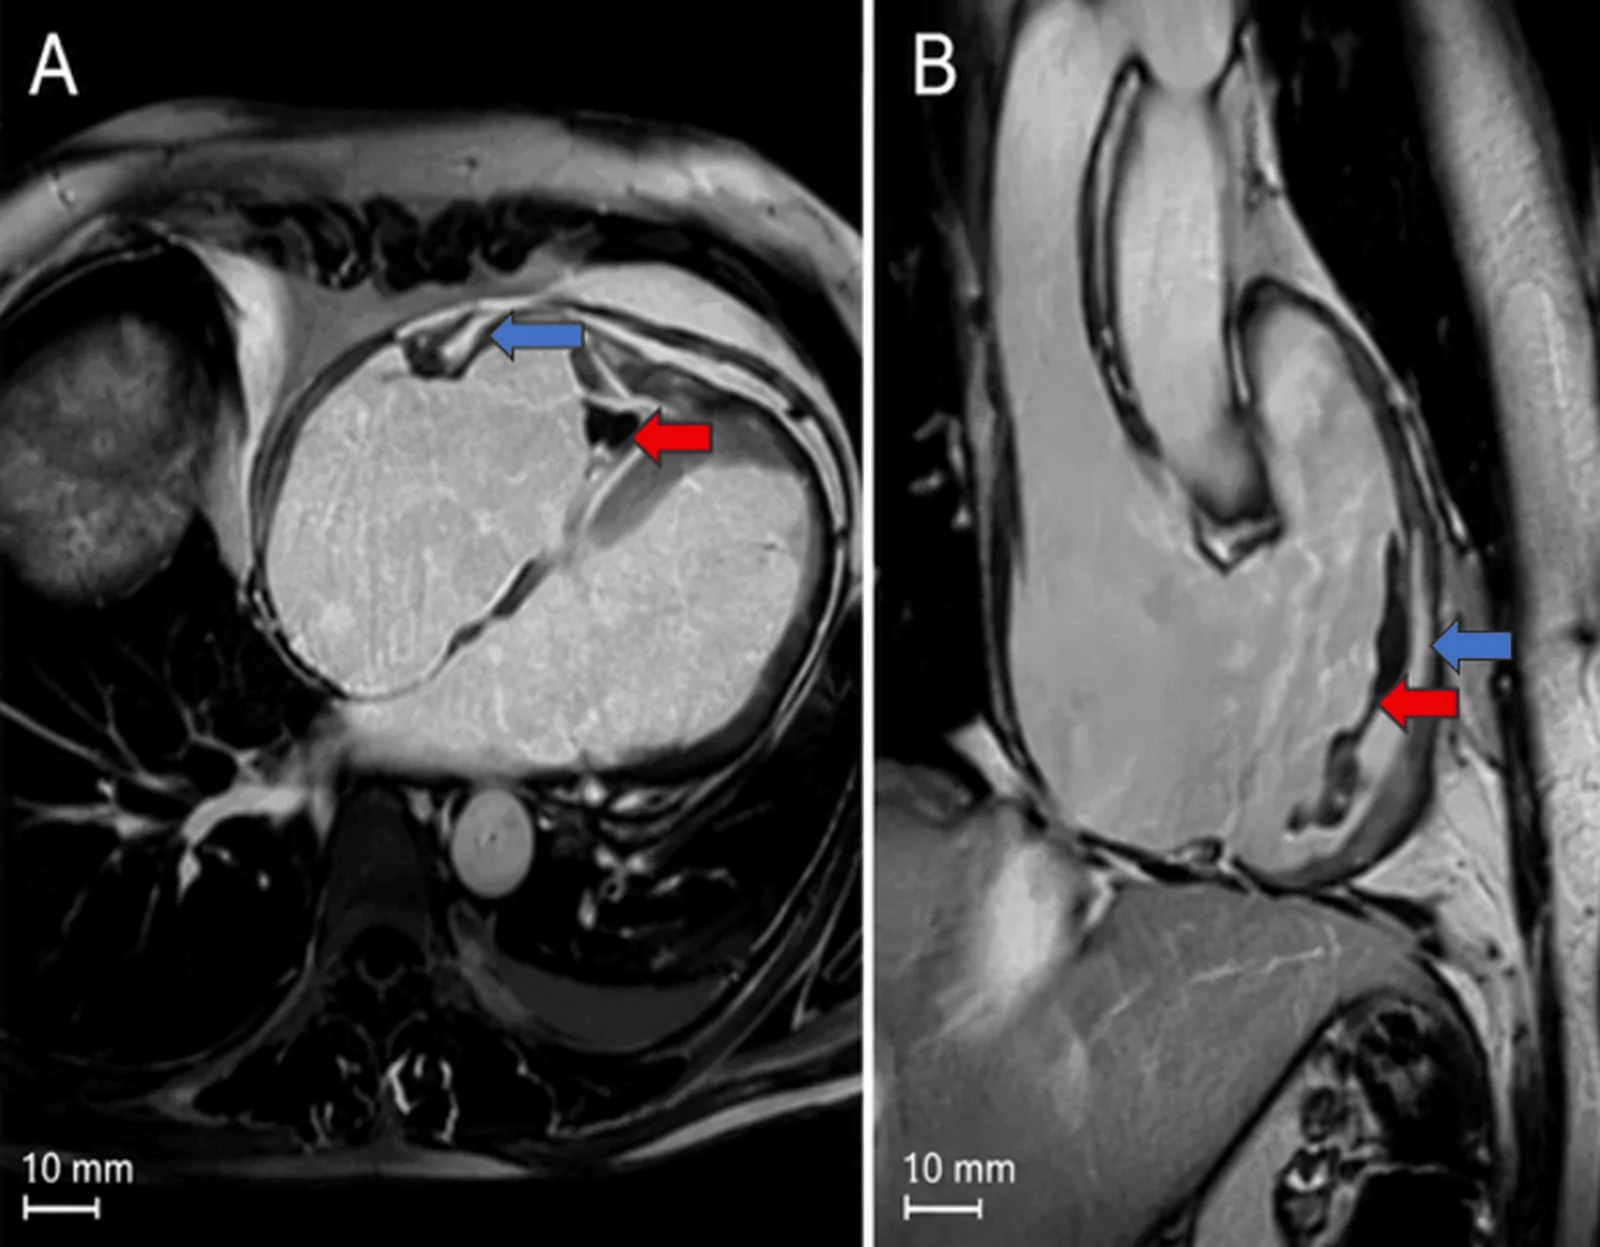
CMR LGE imaging demonstrating isolated right ventricular endomyocardial fibrosis with associated apical thrombus. (A) Apical four-chamber LGE image and (B) right ventricular inflow-outflow LGE image demonstrating diffuse circumferential subendocardial LGE of the right ventricle (blue arrows), consistent with right ventricular endomyocardial fibrosis, associated with an apical intracavitary thrombus (red arrows). CMR, cardiac magnetic resonance; LGE, late gadolinium enhancement
